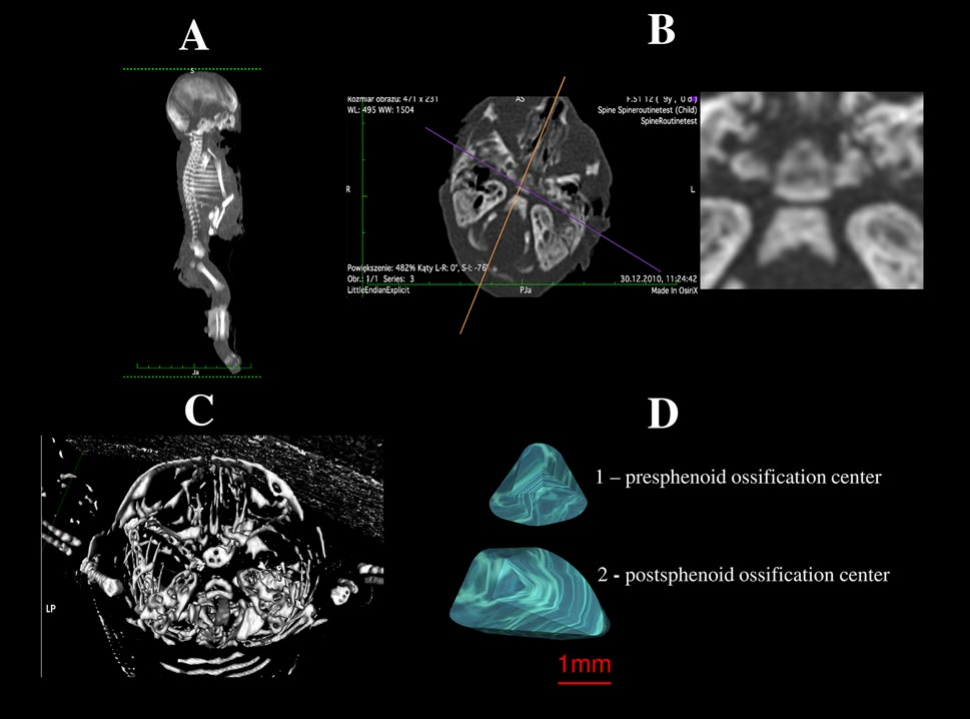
CT of the whole female human fetus aged 26 weeks in the sagittal projection (A) with its cranial CT in the transverse projection (B), CT reconstruction of the presphenoid and postsphenoid ossification centers using Osirix 3.9 MD (C), and volume of the presphenoid (1) and postsphenoid (2) ossification centers (D).
